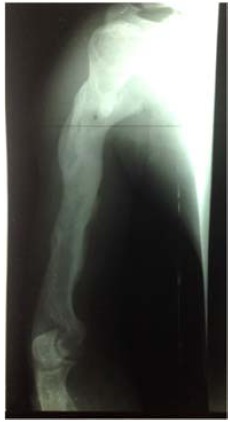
Control x-ray after 48 months.
